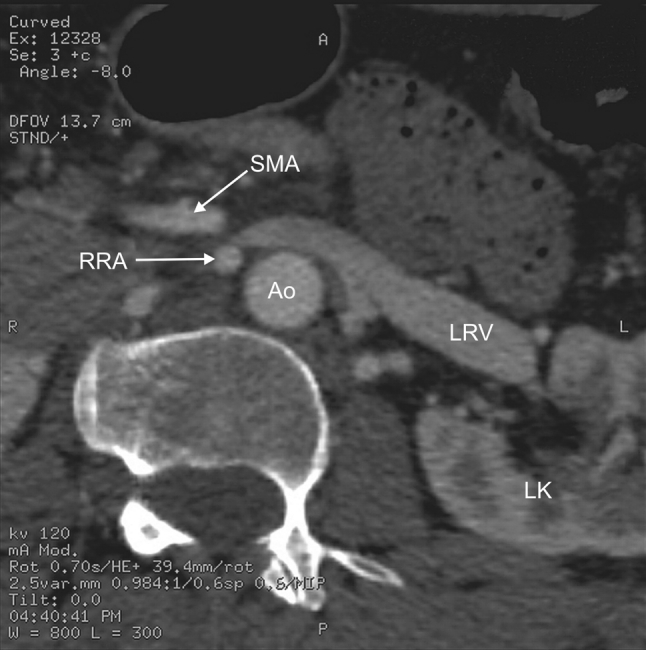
Helical CT angiography, transverse scan at the L2 level. *Ao* abdominal aorta, *LRV* left renal vein, *LK* left kidney, *SMA* superior mesenteric artery, *RRA* right renal artery
